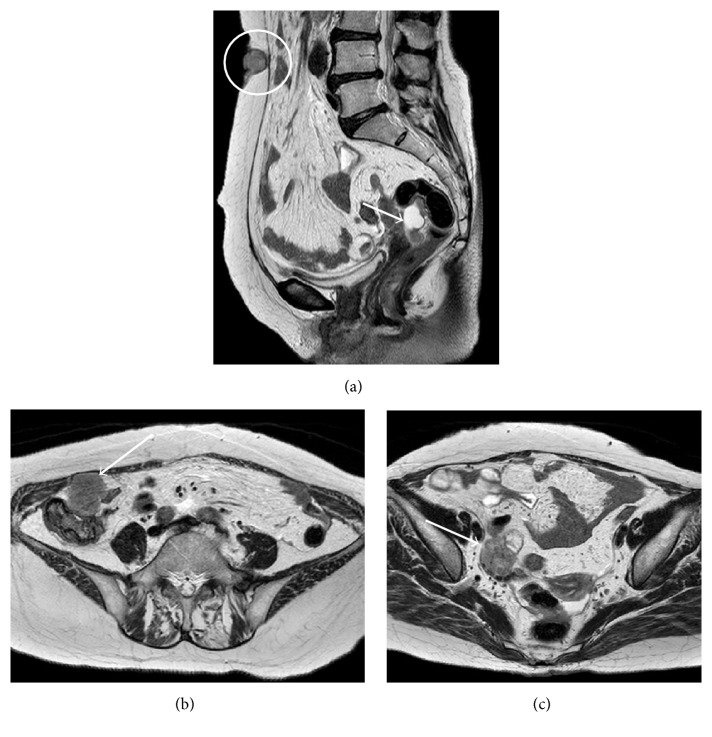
MRI images showed tumors in the ovary (a, →) and umbilicus (a, circle). Also intra-abdominal nodular lesions were noticed (b, c →).
